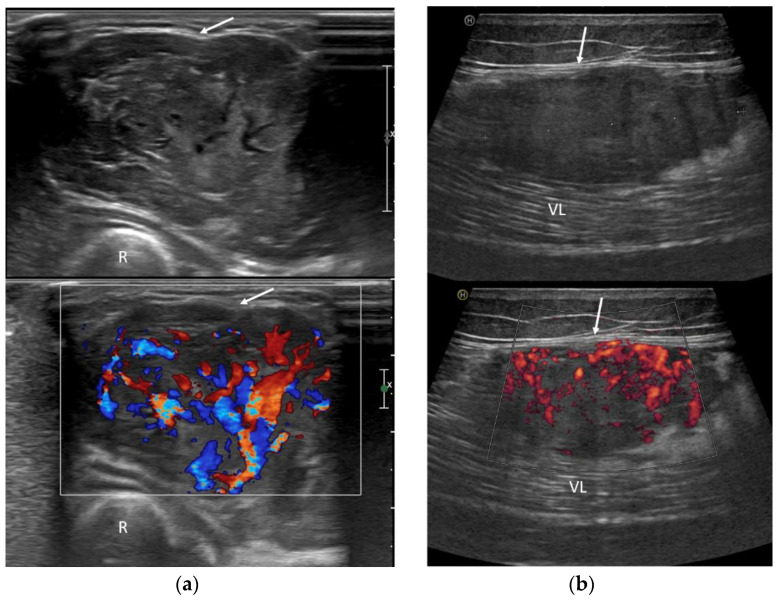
Ultrasound exams in two cases of ASPS demonstrate deep tumor localization in relation to the investing fascia (arrows) and rich neoplastic vascularization on color-Doppler assessment. (a) US images of the forearm (R: radius); (b) US images of the thigh (VL: vastus lateralis muscle).
